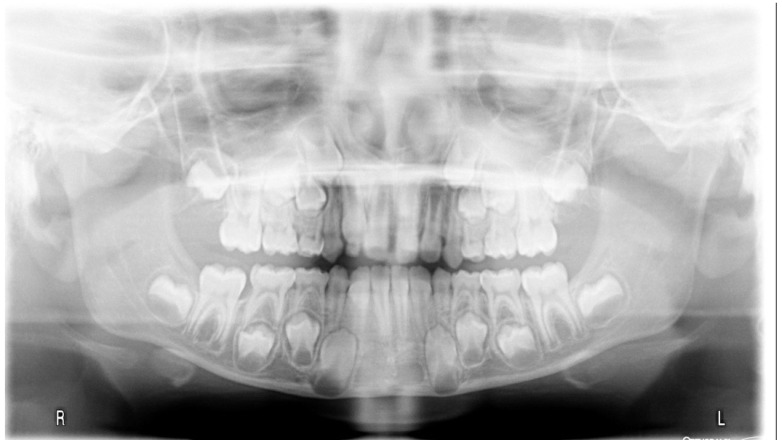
Radiographs of the patient at the screening. Orthopantomography X-ray before treatment (7-year-old).
